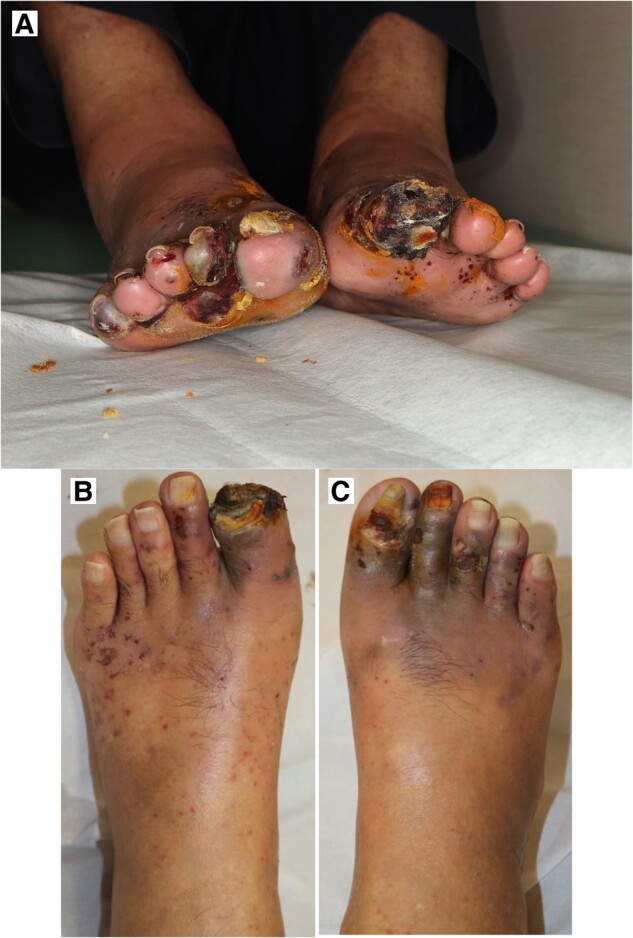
Images of the patient’s lower limbs before low-intensity pulsed ultrasound treatment (*A–C*). The ankle-brachial indexes were 1.14 on the right limb and 0.91 on the left limb with a visual analogue scale of 85.
